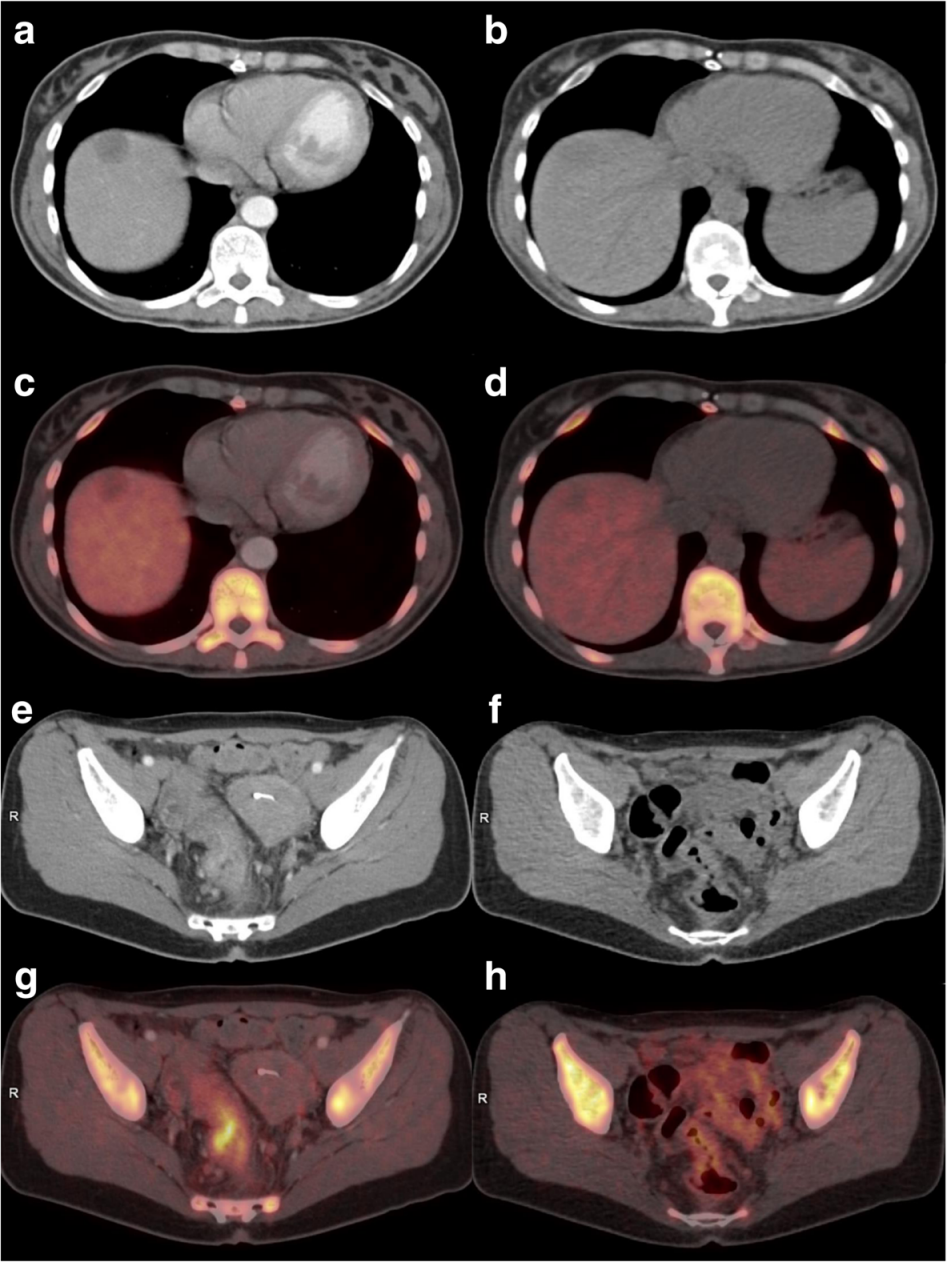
Left column images* show baseline scanning, and *right column* shows images from the evaluation. a A metastasis is seen on the CT as a hypodense focus in the right liver lobe. c The corresponding PET/CT reveals slight pathological FLT uptake in the liver metastasis and relatively high physiological FLT uptake in the normal liver parenchyma. b The metastasis is more hypodense at the time of evaluation and the corresponding PET/CT. d Decreased FLT uptake as a non-active focus in the right liver lobe. e The primary tumour in rectum (baseline) is visualised on CT with the corresponding PET/CT. g High FLT uptake in the tumour. Physiological high FLT uptake is seen in the bone marrow and in the normal intestine. There is no uptake in the uterus in the left side of the pelvis. f At the evaluation, structural shrinkage of the primary tumour on CT is shown and the corresponding PET/CT. h Normalised FLT uptake in the residual tumour. There is physiological FLT uptake in the bone marrow and in the small intestine in the left side of the pelvis
